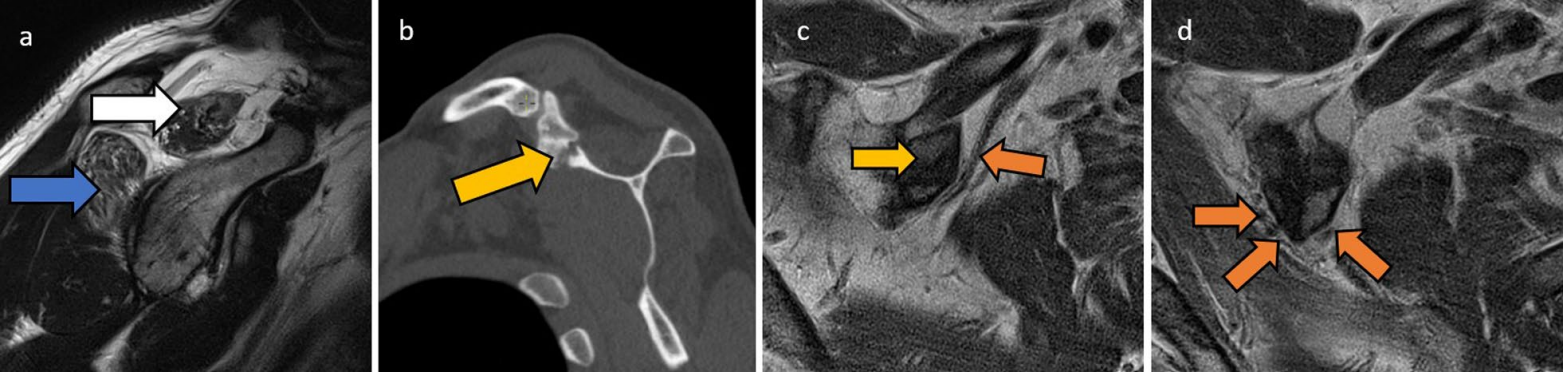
49-year-old male with right upper limb weakness. Sagittal PD (a) shows moderate-severe fatty atrophy of the supraspinatus (white arrow) and infraspinatus muscles (blue arrow) which is compatible with suprascapular nerve entrapment. Interestingly, the site of the impingement is not in the typical suprascapular groove, but more proximally, secondary to ossification along the coracoclavicular ligament which is seen on sagittal CT (b, yellow arrow). The patient had a history of acromioclavicular sprain. Consecutive axial PD images (c, d) show that the ossification (c, yellow arrow) contacted and displaced the suprascapular vessels and nerve (c, d, orange arrows) posteromedially, stretching the nerve
